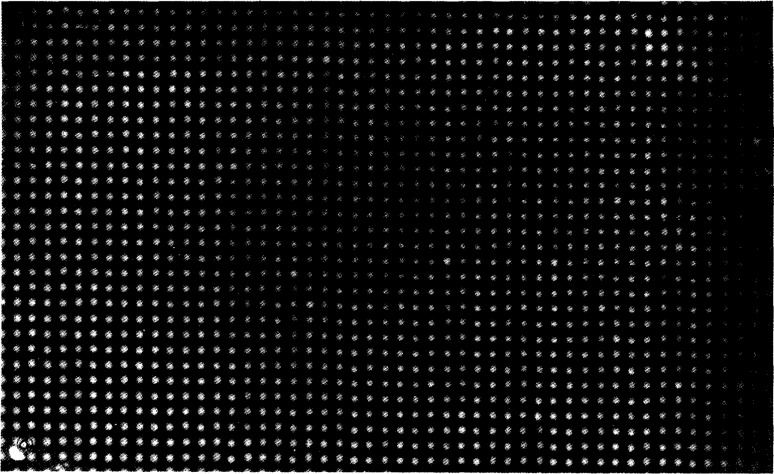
Radiograph of the large area source showing an array of radioactive sites.
